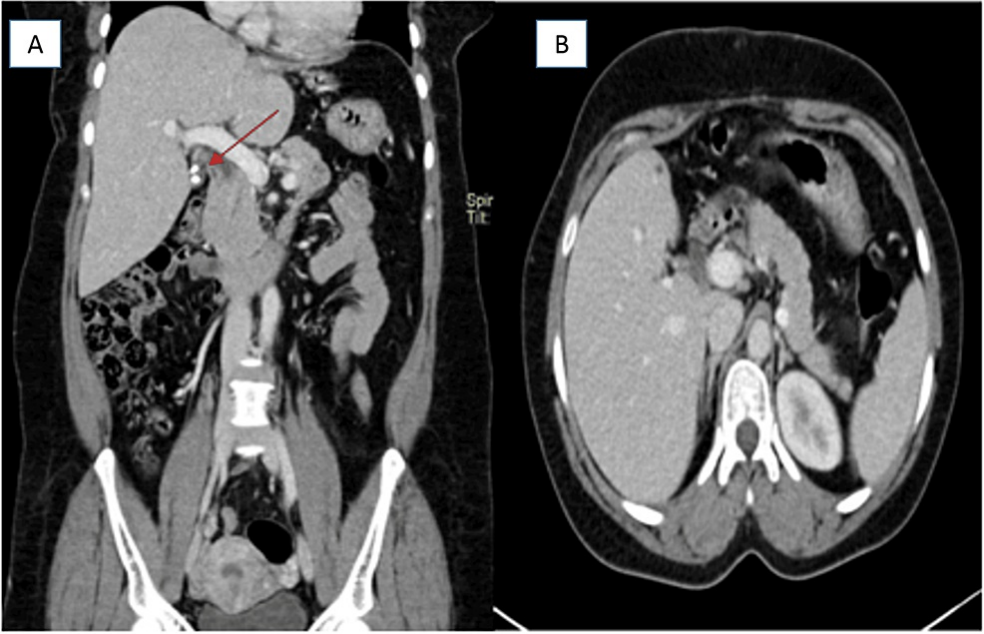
CT scan of the abdomen with IV contrast (Case 3) CT scan of the abdomen with IV contrast (A: coronal plane, B: axial plane). Post antifungal treatment and cholecystectomy show resolution of the porta hepatic mass and cholecystectomy surgical clips (red arrow).
